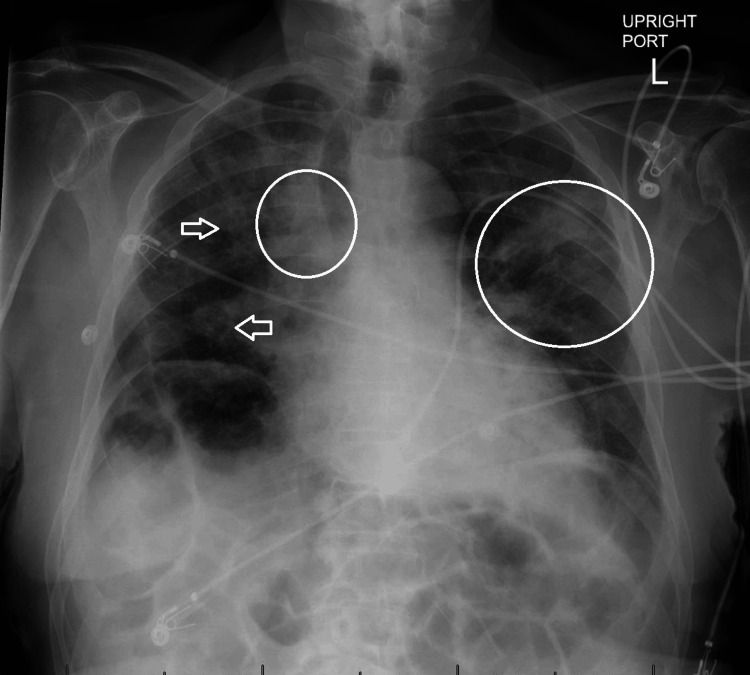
Anteroposterior chest radiograph identifying the location of consolidations (circles) and bronchial wall thickening (arrowheads).
